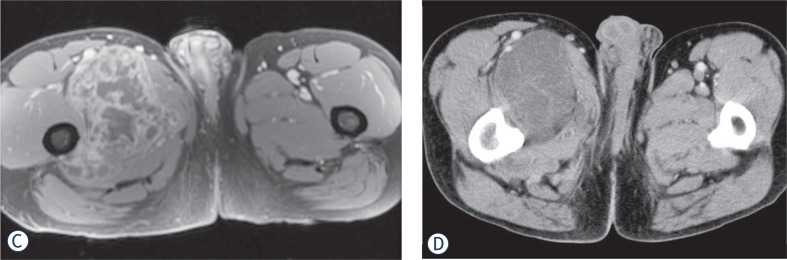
Axial T1 FSPGR FS post-contrast MRI demonstrates peripherhal/septal contrast enhancement. (D). Axial contrast enhanced CT shows that this mass is slightly hypodense to muscle.
